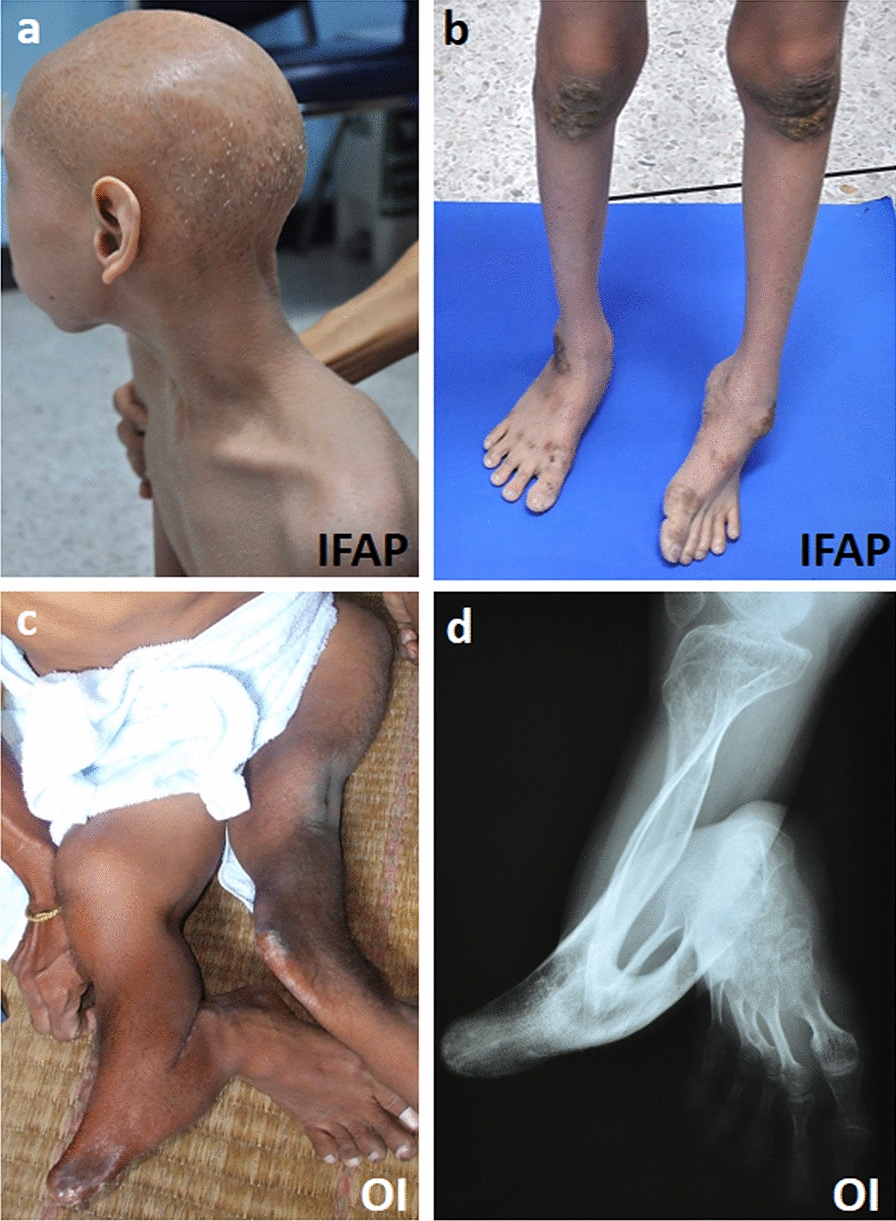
Clinical and radiographic images of patients with IFAP or OI caused by *MBTPS2* mutations. a, b A patient with IFAP harboring the p.R429H mutation in *MBTPS2* exhibited alopecia, ichthyotic scaling, scaly hyperkeratotic plaques over scalp, hyperkeratosis, and dystrophic nails c, d A patient with OI harboring p.N459S mutation in *MBTPS2* exhibited a severely anterior angulation of legs
